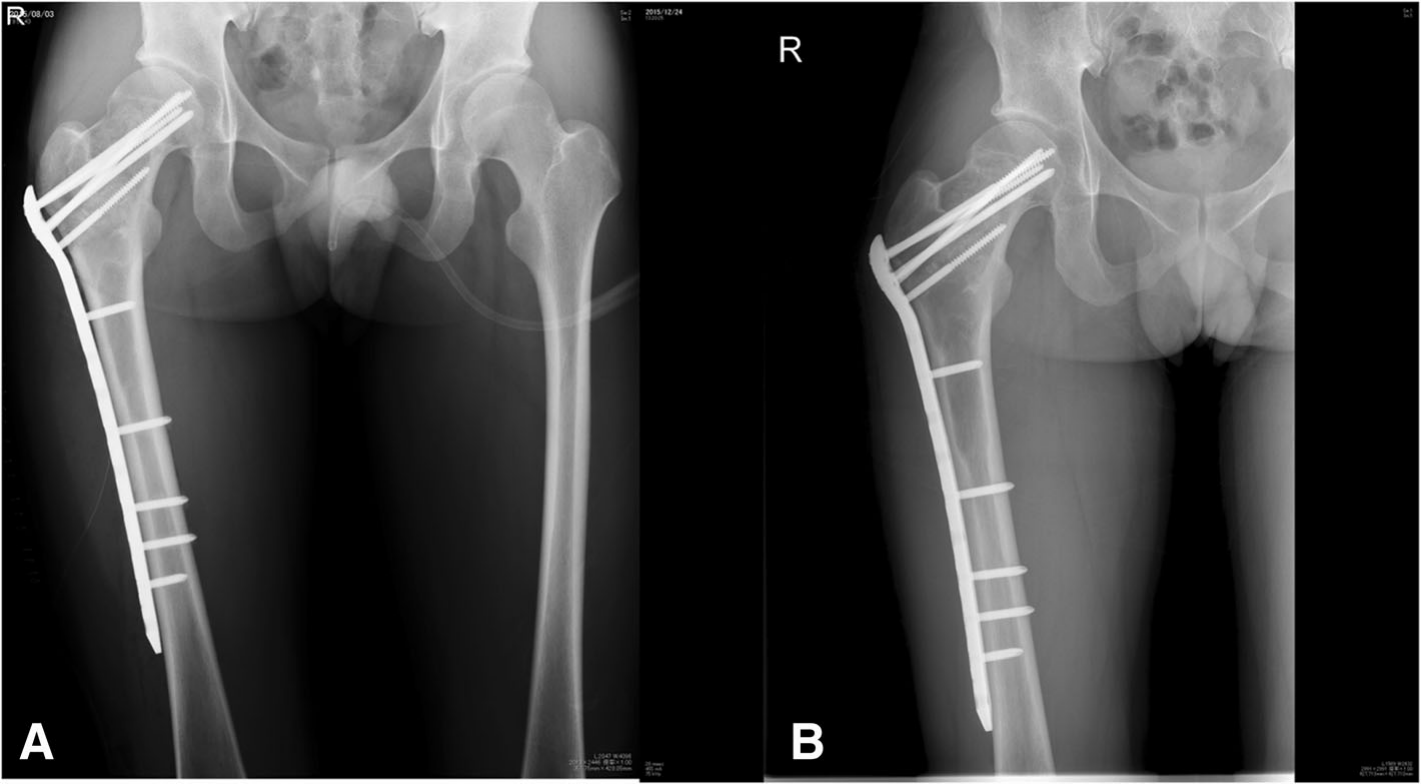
Patient 12: a AP radiograph immediately after prophylactic plating of proximal femoral lesion with proximal femoral locking plate (note proximal screws inserted through the lesion). b Postoperative AP radiograph at 18 weeks showing progression of the lesion
